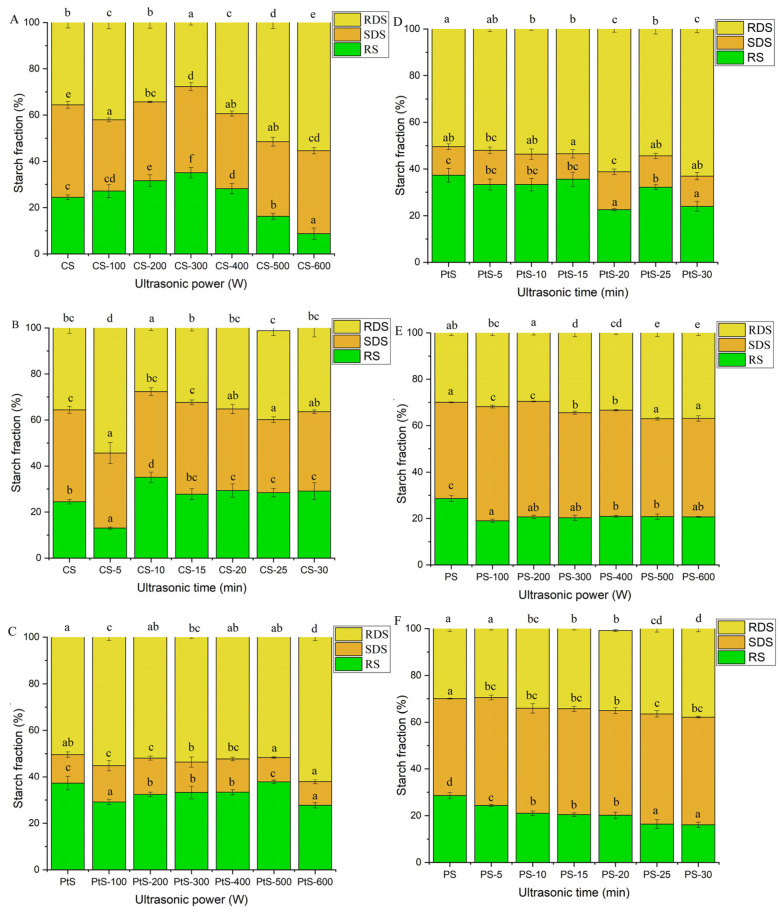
Digestion profile of native and sonicated corn, potato and pea starches under different ultrasonic powers and times; (A,B) native and sonicated corn starch (CS); (C,D) native and sonicated potato starch (PtS); (E,F) native and sonicated pea starch (PS). Different letters upon the pillars of the same color indicate statistical differences (*p* < 0.05) [71].
